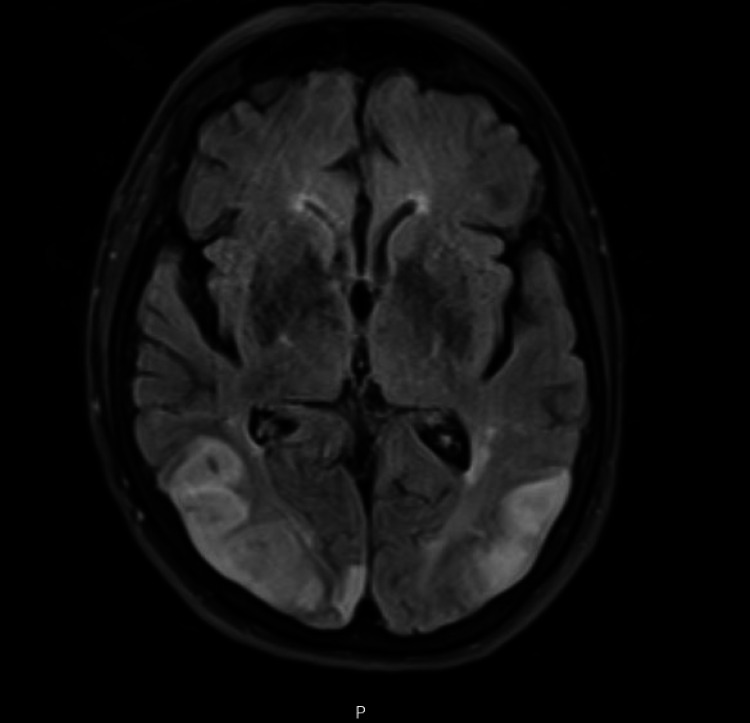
MRI brain showing diffuse abnormalities of bilateral posterior temporal lobes consistent with acute ischemia.
